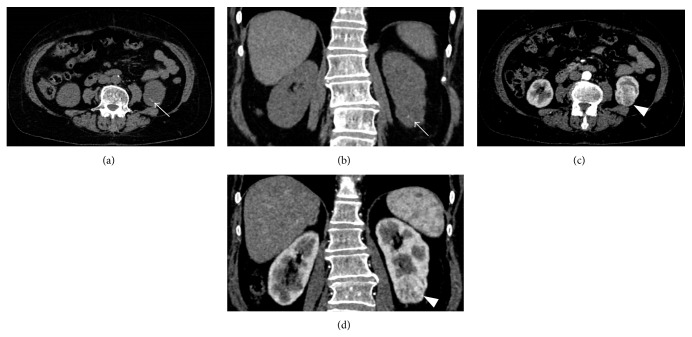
Clear cell carcinoma. ((a)-(b)) Unenhanced CT scans. Small calcifications are recognizable at the lower pole of the left kidney (arrows). ((c)-(d)) Contrast-enhanced CT scans. The lower polar tumor is well evident (arrowheads).
